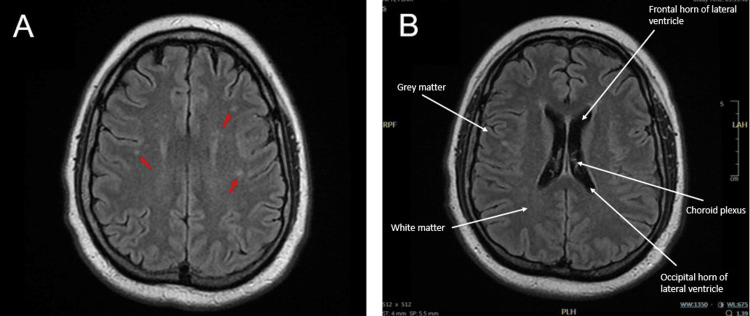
Axial T2 FLAIR MRI images of the brain and orbits revealed minimal white matter lesions characteristic of demyelinating disease. Figure A demonstrates some subcortical white matter hyperintensity (red arrows), but is otherwise unremarkable. Like Figure A, Figure B shows an axial cross-section of the brain with no evidence of hydrocephalus or other acute intracranial abnormality. FLAIR: fluid-attenuated inversion recovery.
